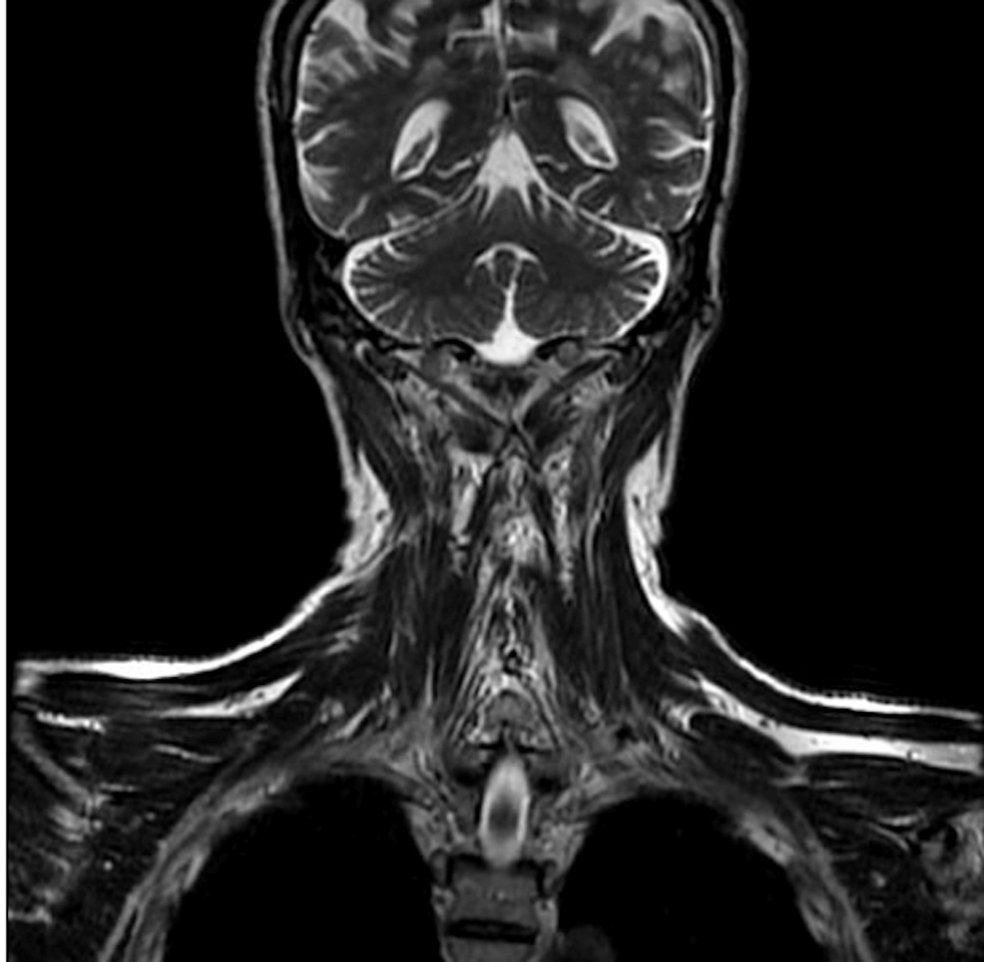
MRI head and neck T2-weighted MRI of head and neck coronal sections showed no discrete lesions MRI: magnetic resonance imaging
